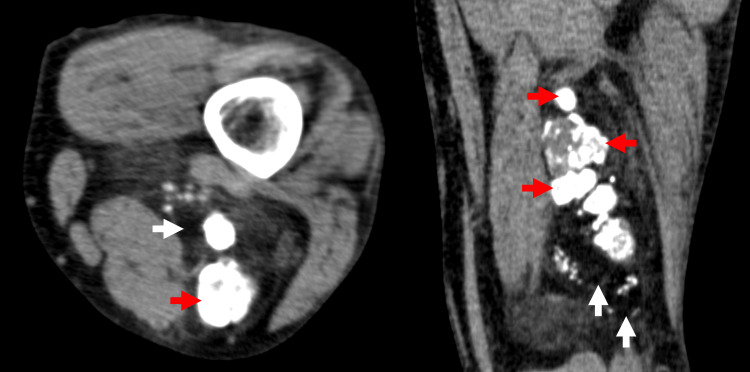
CT images during the CT-guided biopsy Axial (a) and coronal (b) CT images taken during the CT-guided biopsy demonstrate a mass containing both calcified (red arrows) and fatty areas (white arrows). The calcified components have enlarged compared to the previous PET/CT images performed at the referring hospital. PET/CT: positron emission tomography/computed tomography
